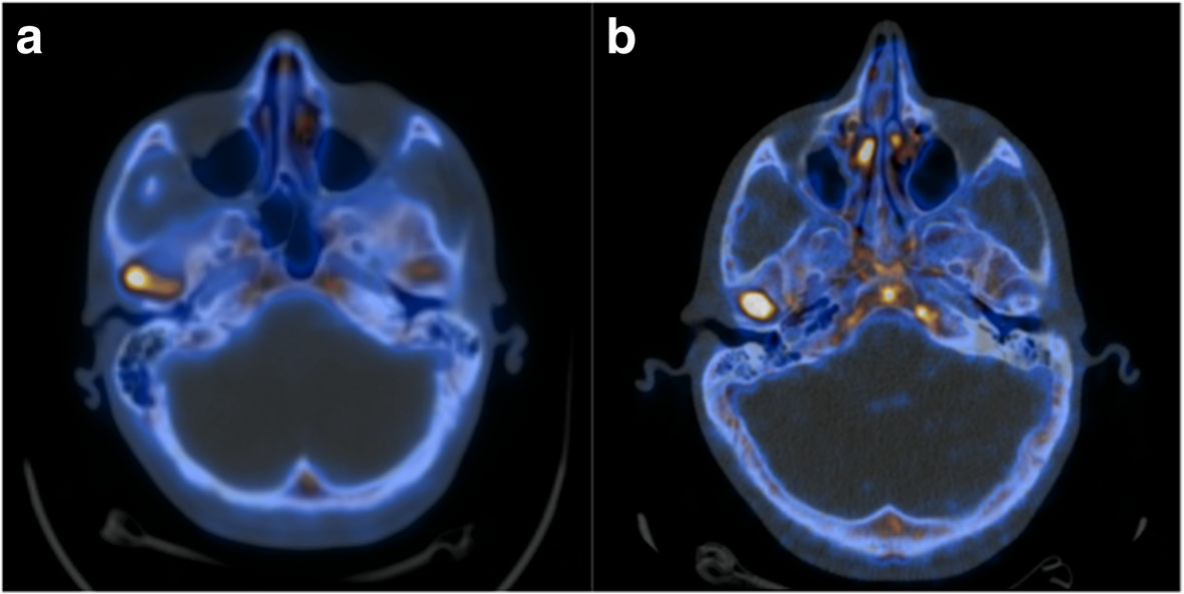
a PET/CT scan performed with an ordinary radiotracer activity [5.3 MBq/Kg of 18F–NaF]; b PET/CT scan performed with a low radiotracer activity [2.9 MBq/Kg of 18F–NaF]
